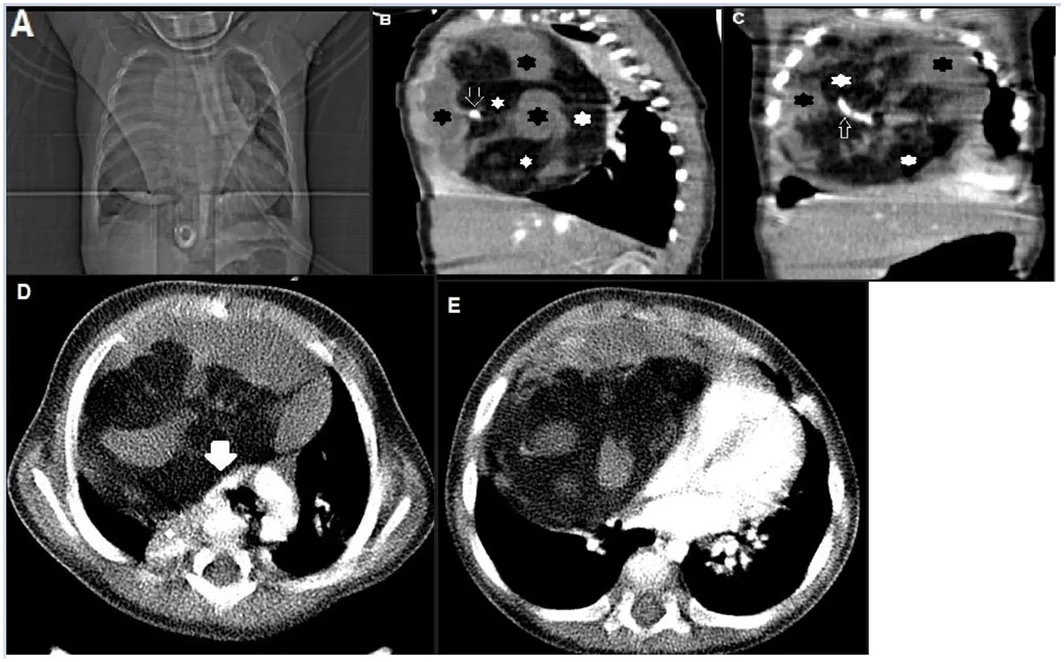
Contrast‐enhanced computed tomography (CT) of the chest. Scout image (A) shows a large mediastinal mass. Sagittal (B) and coronal (C) reconstructed images show a large anterior mediastinal mass with fat (white stars), cystic (black stars), and calcified (white empty arrows) components, compatible with teratoma. Axial images (D, E) demonstrate compression of the superior vena cava (SVC; white solid arrow in D) and the right heart (E). Collapse of the posterior segment of the right upper lobe (RUL; white solid diamond) is evident due to compression of its segmental bronchus.
